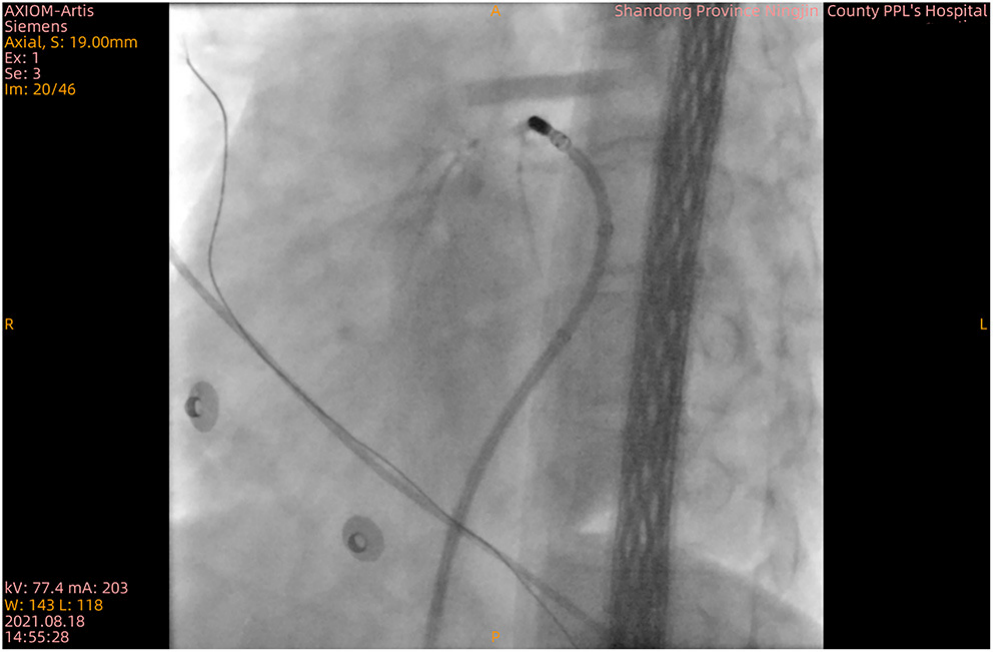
X-ray confirming blood circulation in the great cardiac vein.
